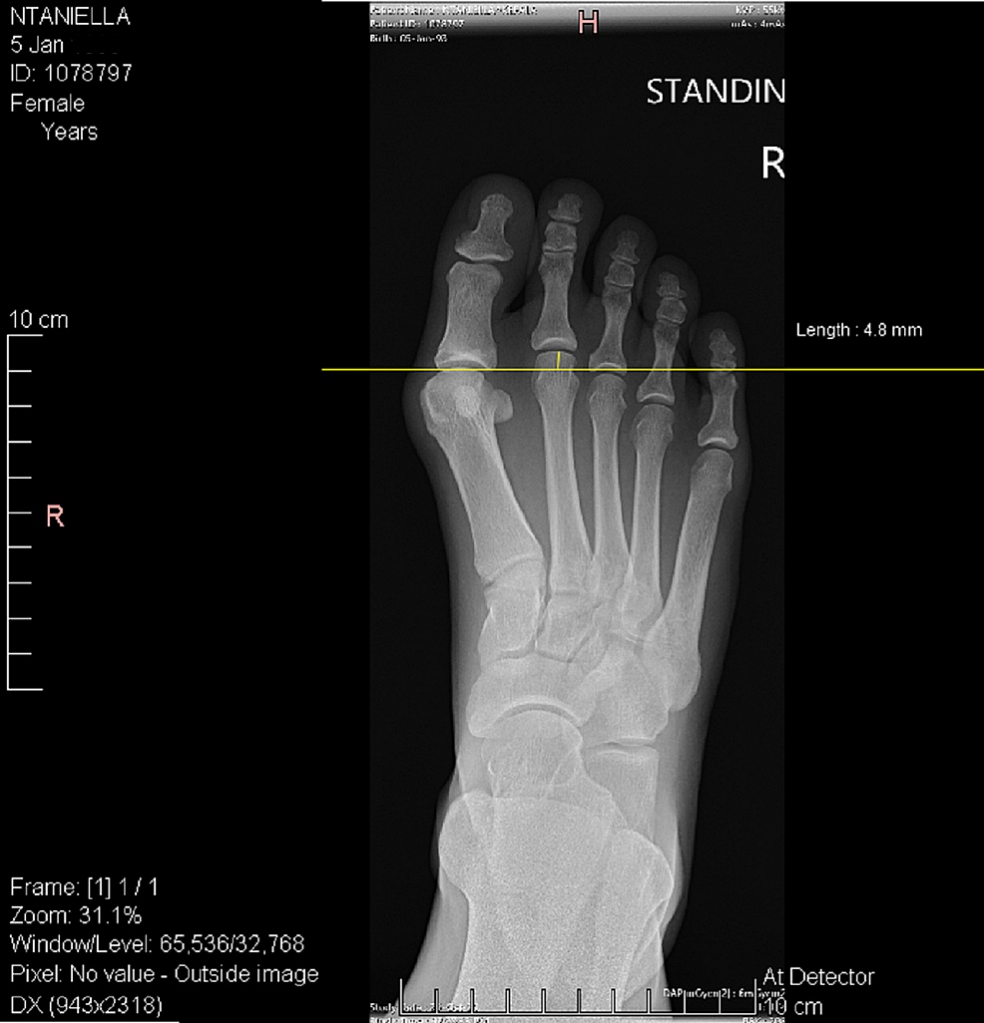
Preoperative anteroposterior weight bearing X-ray of a 28-year-old female patient which suffered by hallux valgus and third-rocker metatarsalgia due to a long second metatarsal. In this patient a Chevron osteotomy on the first metatarsal and a triple Weil osteotomy on the second metatarsal, were planned. The choice of Maceira’s technique was based on the amount of desired shortening (4.8 mm).
